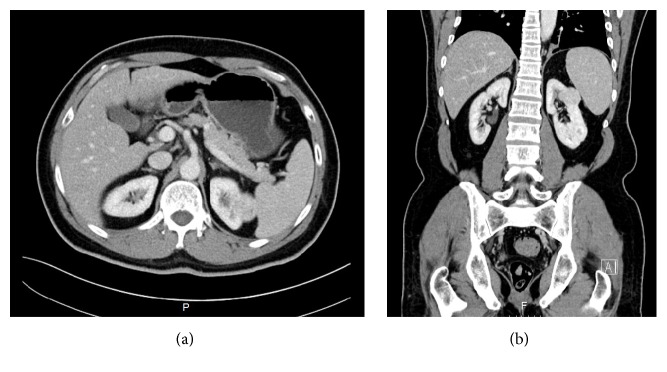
Computed tomography (CT) scan of the abdomen. (a) Axial section and (b) coronal section showing a well-defined heterogeneous lesion measuring 2 × 1.5 cm located at the posterolateral upper pole of the left kidney, most likely arising from kidney cortex.
